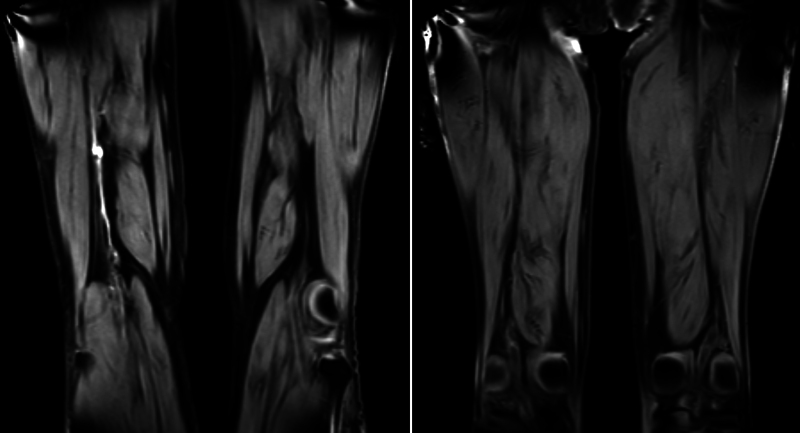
An MRDTI scan indicating acute thrombosis and an MRDTI scan without signs of acute thrombosis. Left: MRDTI scan demonstrating acute thrombosis. A 70-year-old male patient who presented with suspected recurrent ipsilateral DVT of the right leg. CUS revealed thrombosis of the popliteal vein, calf veins, and to the extent assessable, of the superficial femoral vein. MRDTI scan showed asymmetric high signal intensity in the right proximal superficial femoral vein which could be followed up to the popliteal vein and calf veins, indicating acute thrombosis. Right: MRDTI scan demonstrating the absence of acute thrombosis. A 68-year-old male patient who presented with suspected recurrent ipsilateral DVT of the right leg. CUS demonstrated signs of DVT of the right popliteal vein. MRDTI scan showed symmetric low signal intensity along the deep venous system including the femoral vein and popliteal vein of both legs, indicating the absence of acute thrombosis. Abbreviations: MRDTI: magnetic resonance direct thrombus imaging, DVT: deep vein thrombosis, CUS: compression ultrasound.
